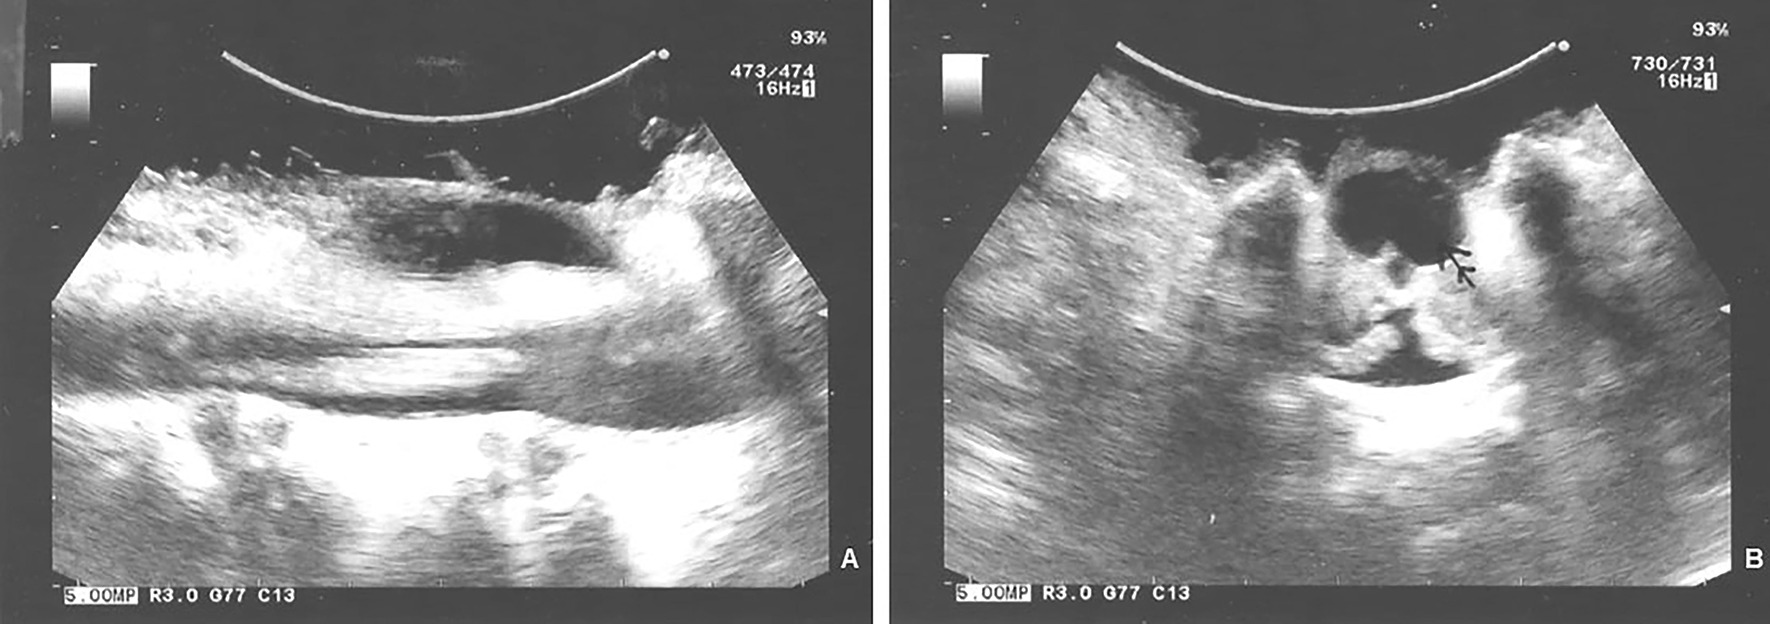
Sagittal (A) and axial (B) intraoperative ultrasound after L1–L3 laminectomies with intact dura. The cyst (arrow) appears to be larger compared with the size demonstrated on magnetic resonance imaging
